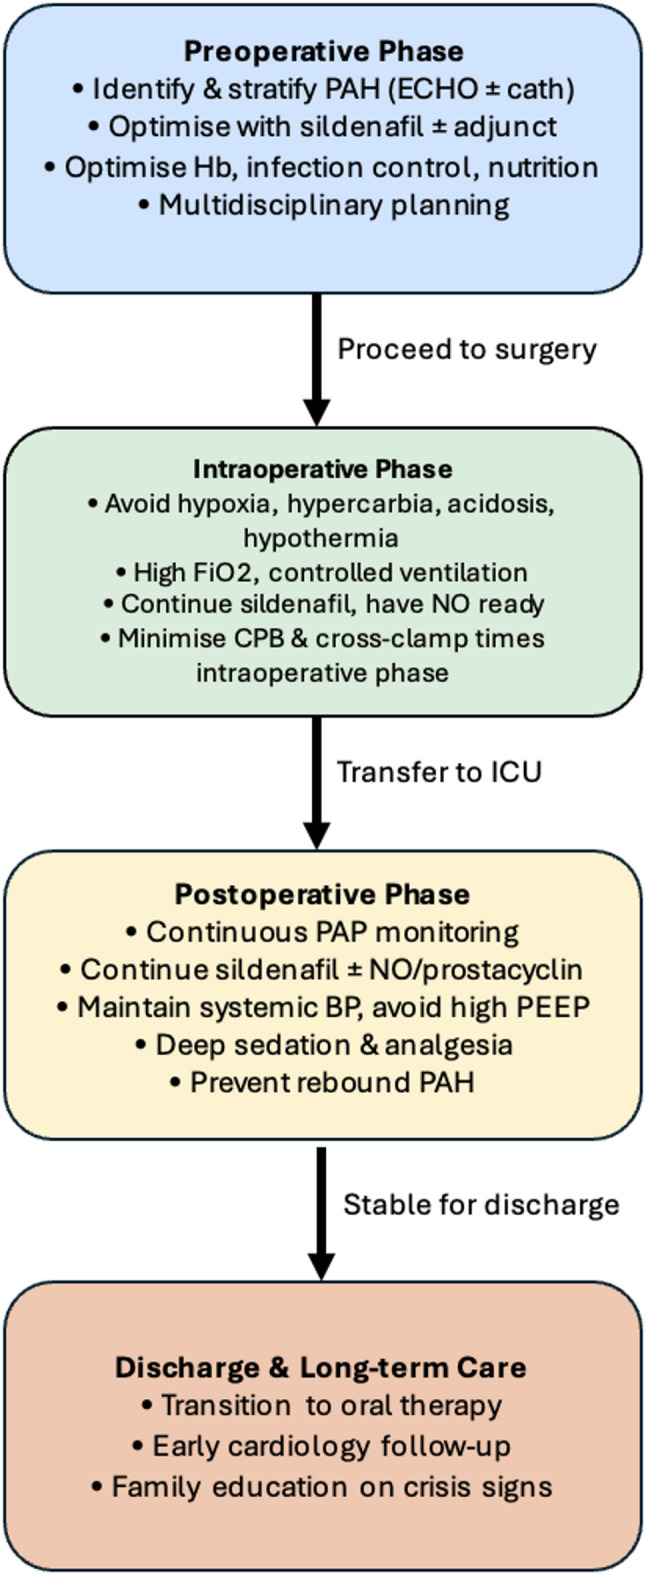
Perioperative management pathway for PAH in children with CHD undergoing cardiac surgery, outlining key interventions from preoperative assessment to discharge; PAH: pulmonary arterial hypertension; ECHO: echocardiography; NO: nitric oxide; CPB: cardiopulmonary bypass; PAP: pulmonary arterial pressure; PEEP: positive end-expiratory pressure
